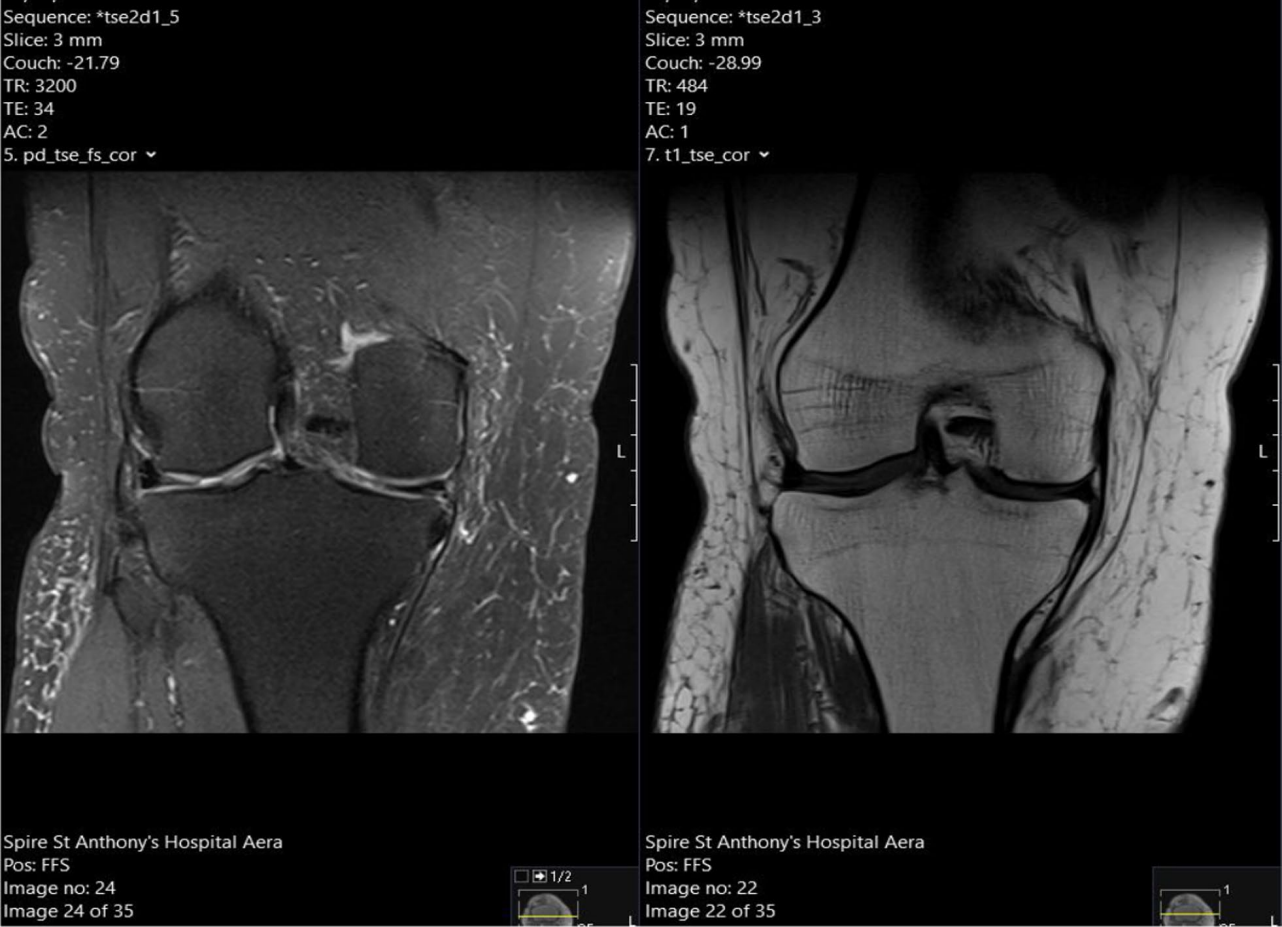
T1 and T2 Magnetic Resonance Imaging scan showing thinning and fragmentation of the articular surface in keeping with osteoarthritis
